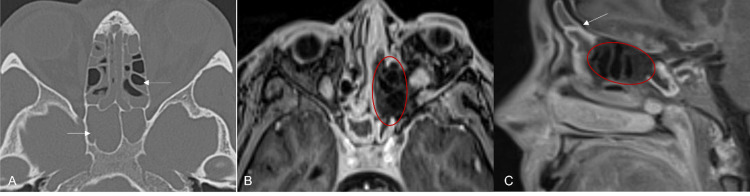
Diffuse sinusitis with necrosis (A) Non-contrast CT bone kernel: diffuse sinus disease centered in the sphenoid and ethmoid sinuses (white arrows); (B) T1 Post-gadolinium MPRAGE: non-enhancing mucosa of left sphenoid sinus (red oval) consistent with necrosis; (C) T1FS post-gadolinium SPACE: partially seen left frontal sinus disease (white arrow) and redemonstration of necrosis of the left ethmoid sinus necrosis (red oval). MPRAGE: magnetization-prepared rapid acquisition gradient echo; T1FS: T1-weighted fat-suppressed; SPACE: sampling perfection with application-optimized contrasts using different flip angle evolution
